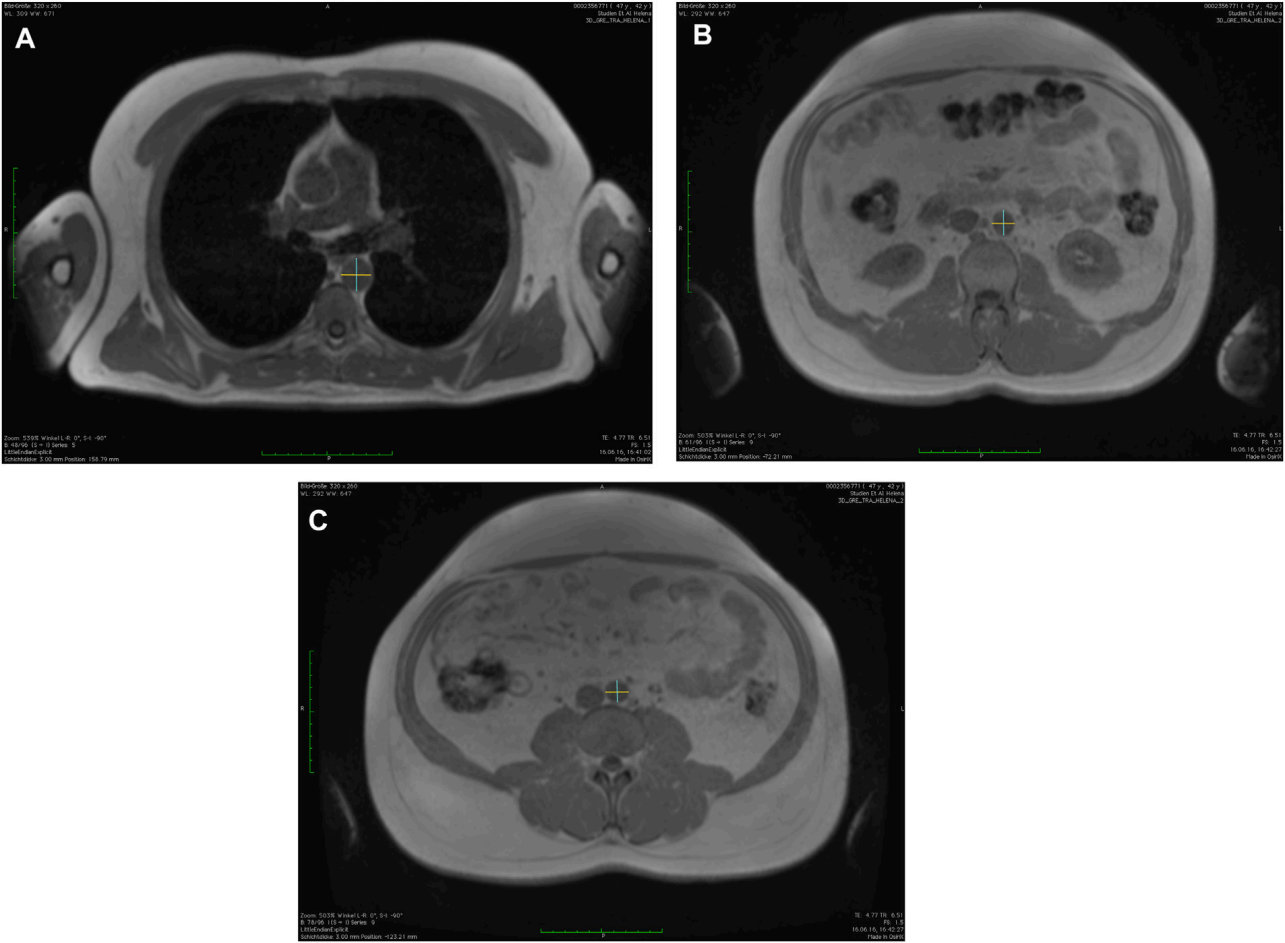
Measurements of the aortic diameters using the in-phase images of the T1-weighted-3D-VIBE two-point Dixon sequence with a post-processing software (OsiriX, Pixmeo SARL, Bernex, Switzerland) in anterior-posterior and left-right orientation at (A) descending thoracic aorta, (B) infrarenal abdominal aorta, and (C) directly above the aortic bifurcation.
